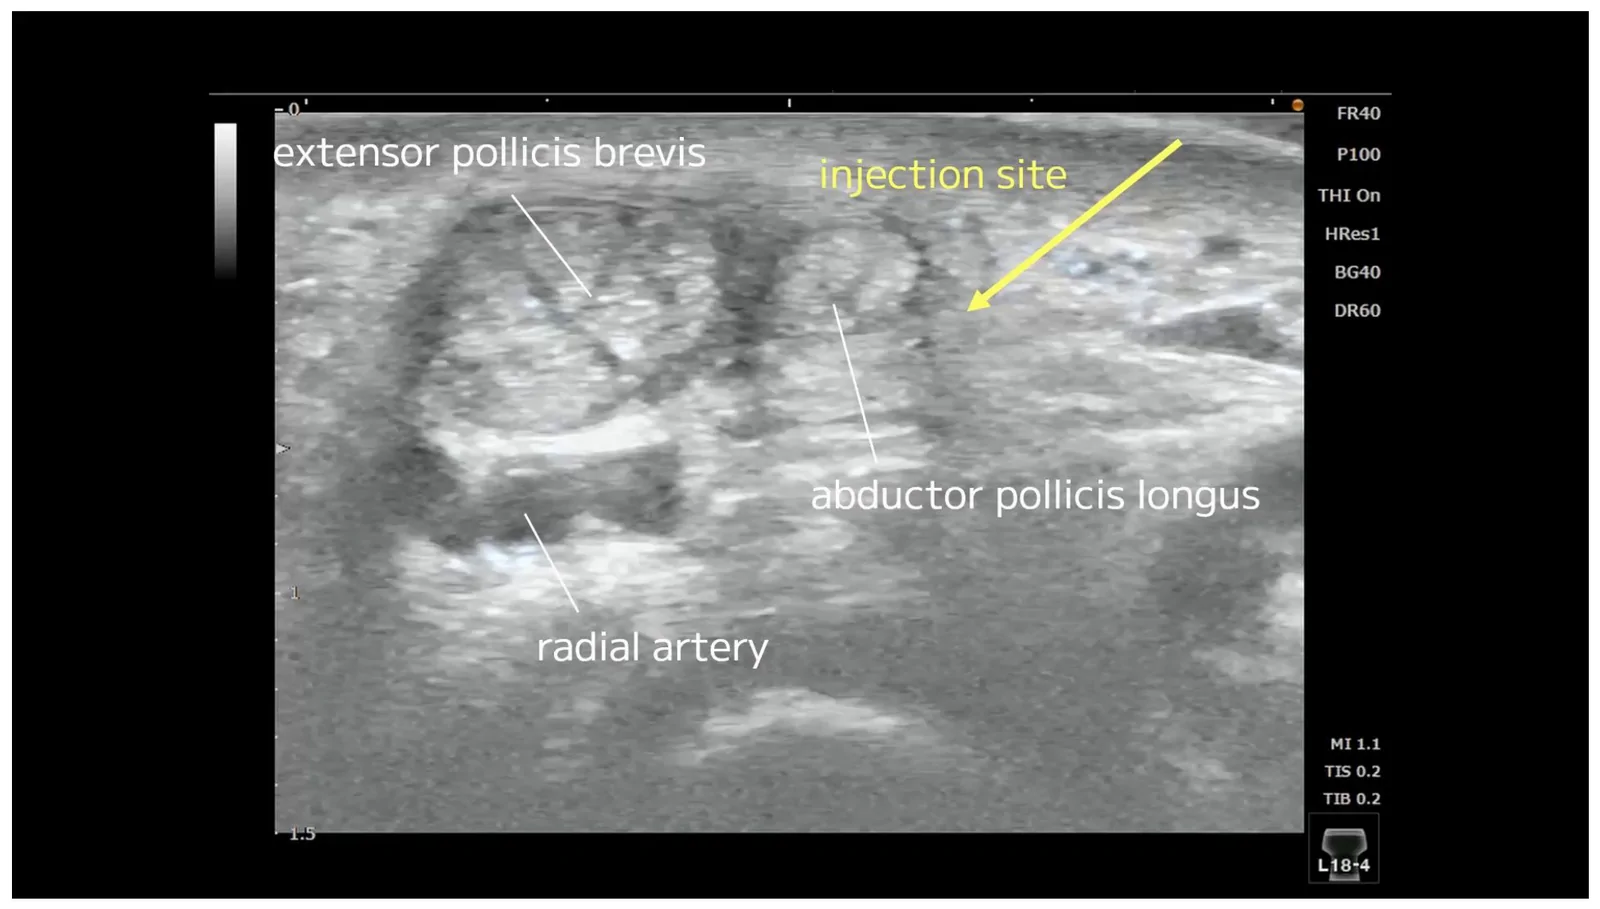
Point 5—radial artery/first compartment crossing: ultrasound-guided fascia hydrorelease; yellow arrow indicates the injection site.
